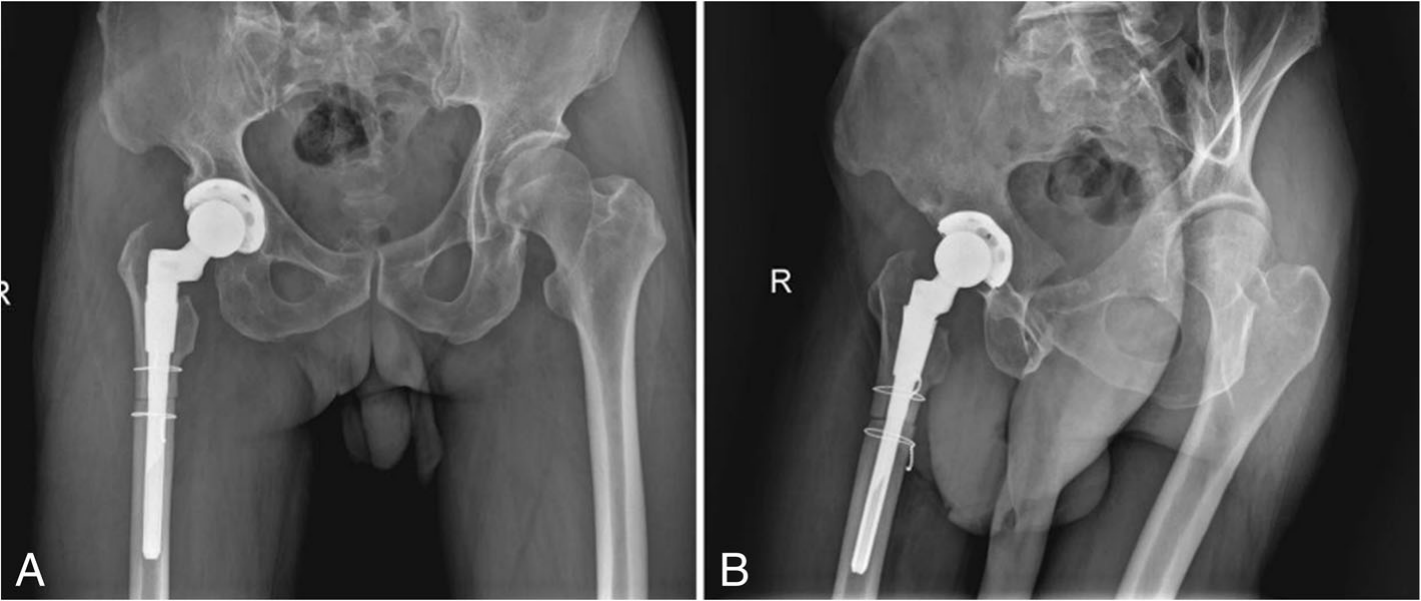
Radiographs of the right hip at 40 days after the operation. (a) anteroposterior view of the right hip, (b) lateral view of the right hip
